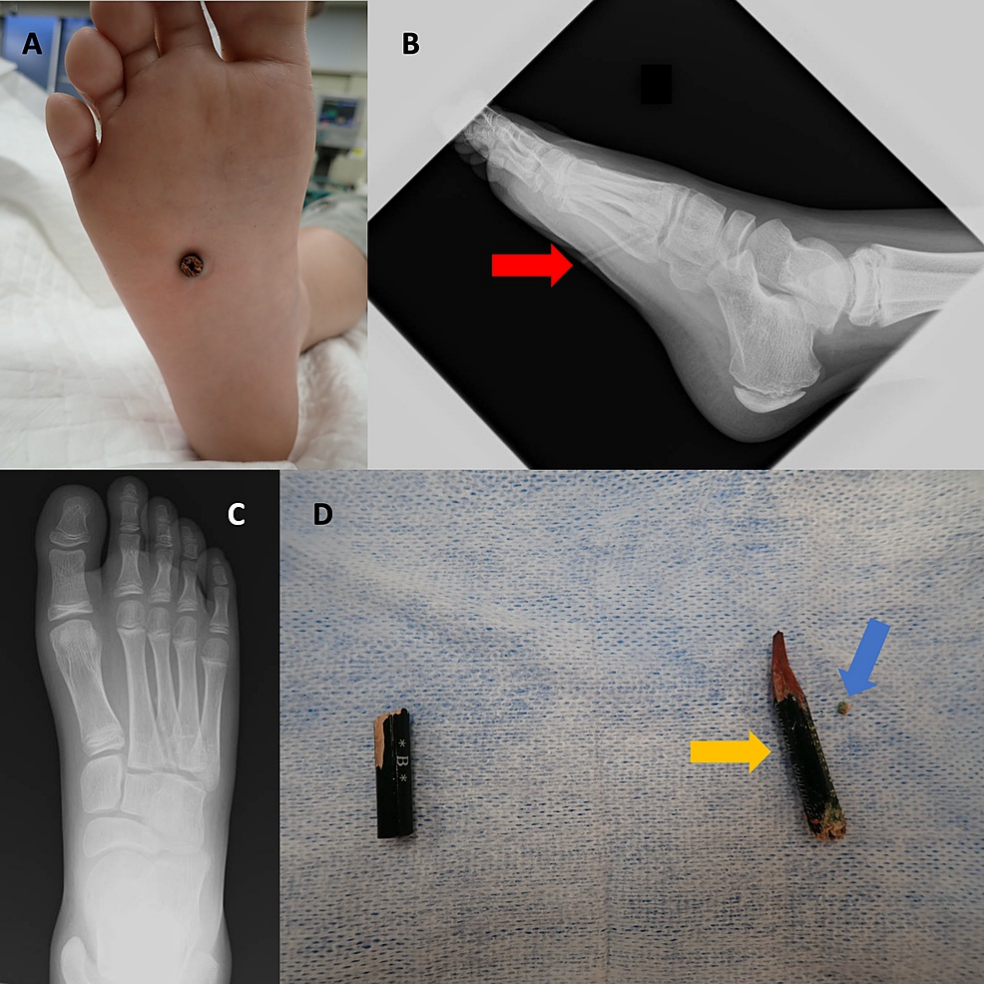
A pencil embedded in a child’s foot. 1A) The end of the pencil was visible on the sole of the right foot; 1B) Lateral X-ray of the right foot. A stick-like foreign body is seen in the right foot (red arrow); 1C) Anteroposterior X-ray of the right foot. The foreign body was not visible; 1D) The fragment on the left is the broken external part of the pencil and the fragment on the right is the part that was embedded in the foot (orange arrow). The smaller right-most fragment is the part that broke off when trying to remove the pencil using mosquito forceps (blue arrow).
